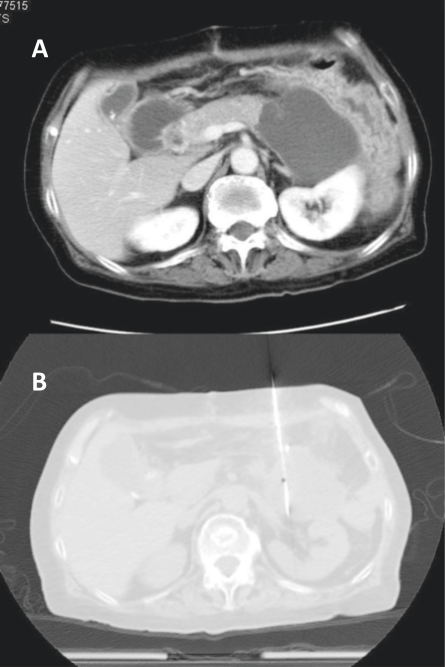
CT on postoperative day 17. (A) CT scan revealed fluid collection at stump of the remnant pancreas. (B) CT-guided drainage was performed. The amylase level of the fluid was 59,676 U/L.
